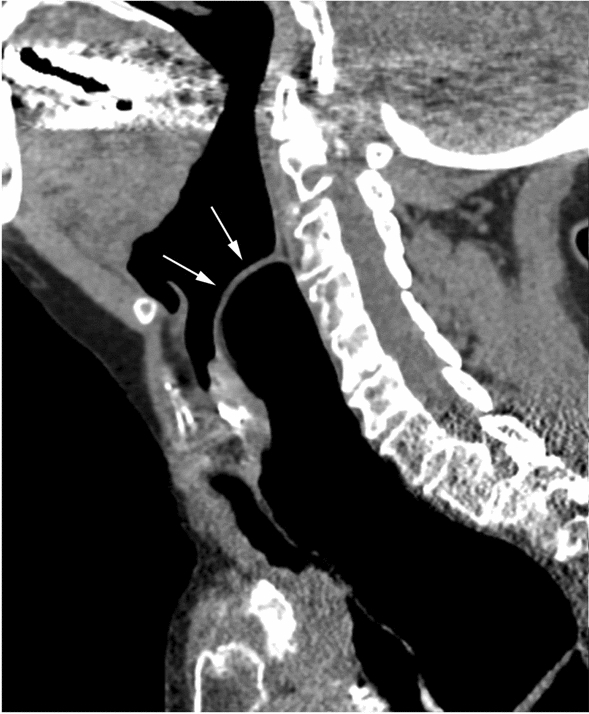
The CT scan of Fig. 4a, here presented after adjusting image contrast settings. The arrows point to the cranial demarcation of the mid-esophageal diverticulum
